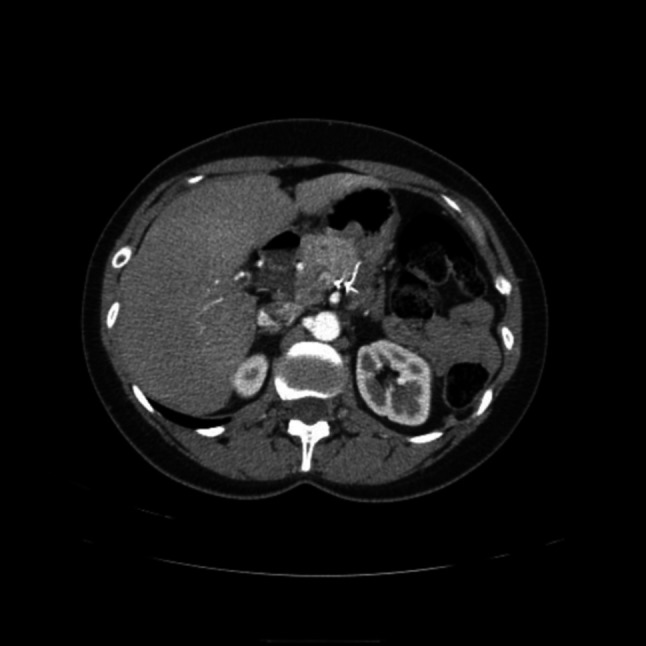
CT scan performed in April 2021, i.e., 6 years and 8 months after stopping somatostatin analog treatment, showing no lesions of the pancreas
